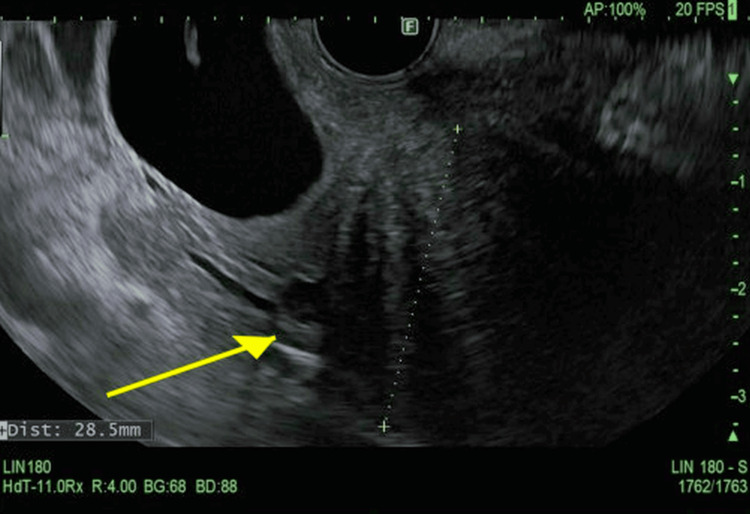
Ultrasound image of the pancreatic mass The dotted line indicates distance measurement of the pancreatic head. The yellow arrow indicates the hypoechoic pancreatic head mass that was targeted with fine needle aspiration
